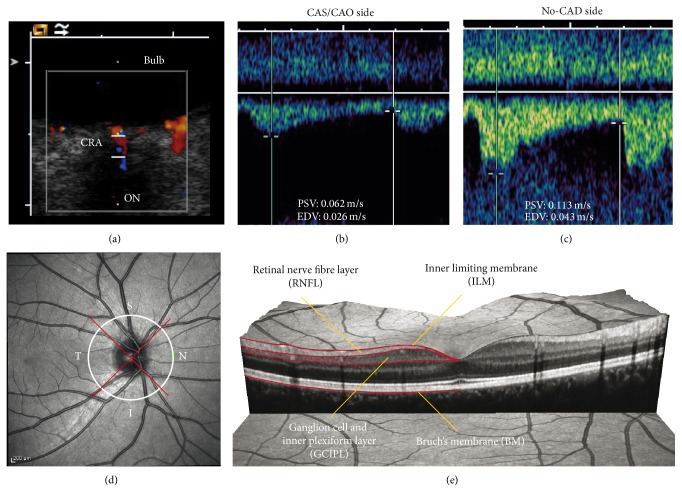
(a)–(c) Transorbital ultrasound measurement of flow velocities in the central retinal artery (CRA) in a patient with carotid artery occlusion (CAO) and retrograde OA blood flow. (a) Transorbital B-mode scan of the optic nerve and color-mode imaging of the CRA. (b) Flow velocities ipsilateral to the CAO. Besides the reduced absolute flow velocity, there is a markedly delayed systolic flow rise. (c) Flow velocity of the no-CAD side. Note the normal steep systolic flow rise. (d) Exemplary peripapillary OCT ring scan with sectors: temporal (T), superior (S), inferior (I), and nasal (N). (e) Exemplary presentation of an OCT macular volume scan with segmentation of the retinal nerve fiber layer (RNFL) and combined ganglion cell and inner plexiform layer (GCIPL).
